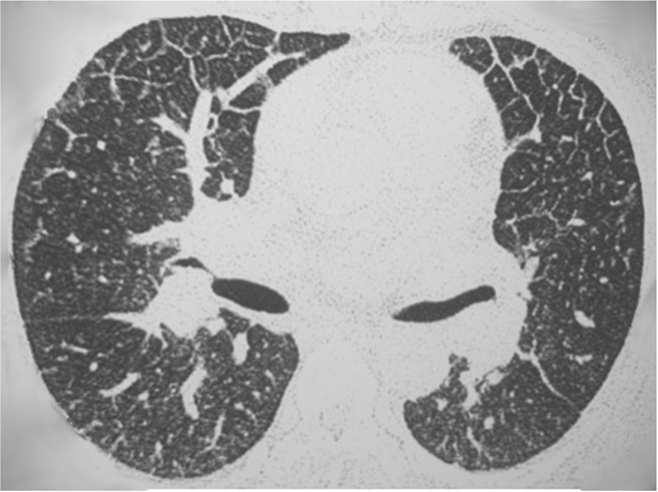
A 38-year-old male with pulmonary toxoplasmosis. Axial view of CT scan displays ground glass opacities and septal thickening.
